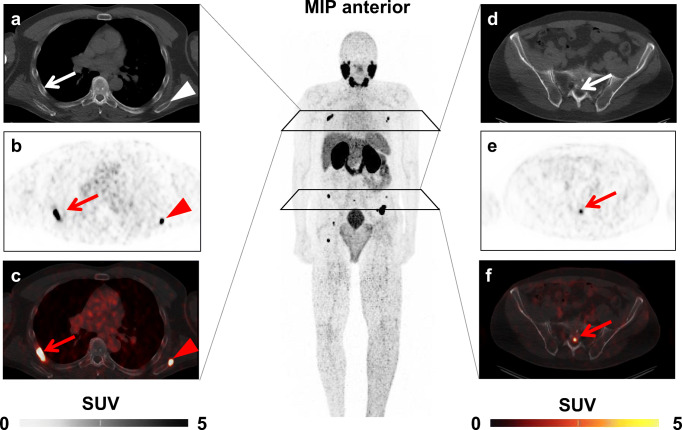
Case example.* Baseline ^68^Ga-PSMA ligand positron emission tomography (PET)/computed tomography (CT) of a 59-year-old patient demonstrating several bone metastases, exemplified by PSMA-avid lesions in the sixth right rib (*arrow*) and left scapula (*arrowhead*) on a CT (without correlate), b PET, and c fused PET/CT images, and in the sacrum (*arrow*) (d–f). Baseline leukocyte count was 4.6 × 10^3^/mm^3^ which was below the receiver operating characteristics derived cut-off of 5.6 × 10^3^/mm^3^ (indicative of elevated risk of leukocytopenia). Osseous tumor volume (TV) was 12.01cm^3^, which was below the cut-off of 100cm^3^ and thus, PSMA-TV in the skeleton would suggest a reduced risk of hematological events. This patient, however, showed grade 1 leukocytopenia after one cycle of radioligand therapy. *MIP* maximum intensity projection, *SUV* standardized uptake value
